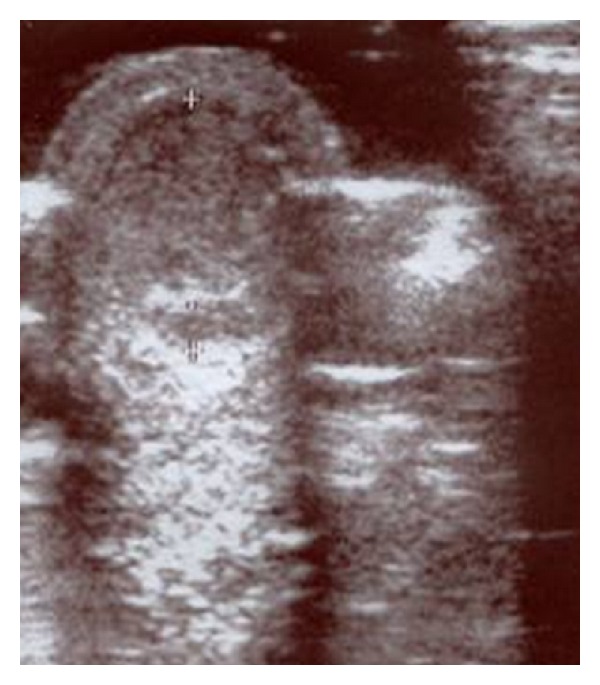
Ultrasound examination showing the presence of the omentum into the hernia.
